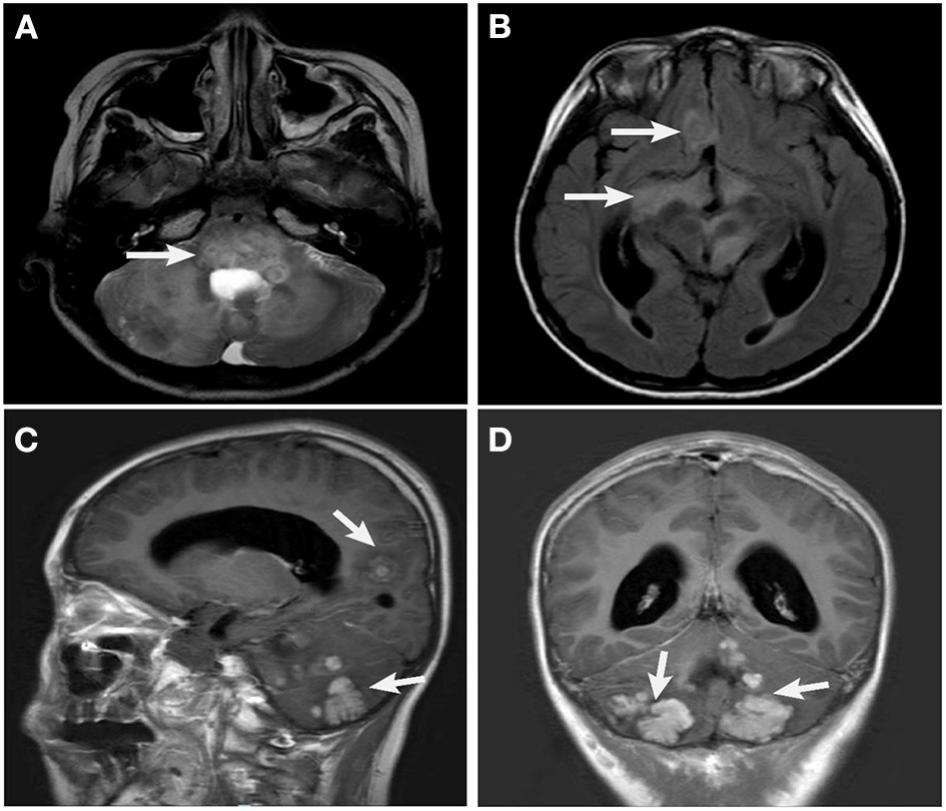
Head MRI (case 10): Brainstem (A), optic pathway, sellar region, anterior middle cranial fossa (B) and cerebellum (C,D), multiple space occupying lesions with slightly longer T2 signal, high signal intensity on FLAIR image, and uneven signal intensity of the lesion.
